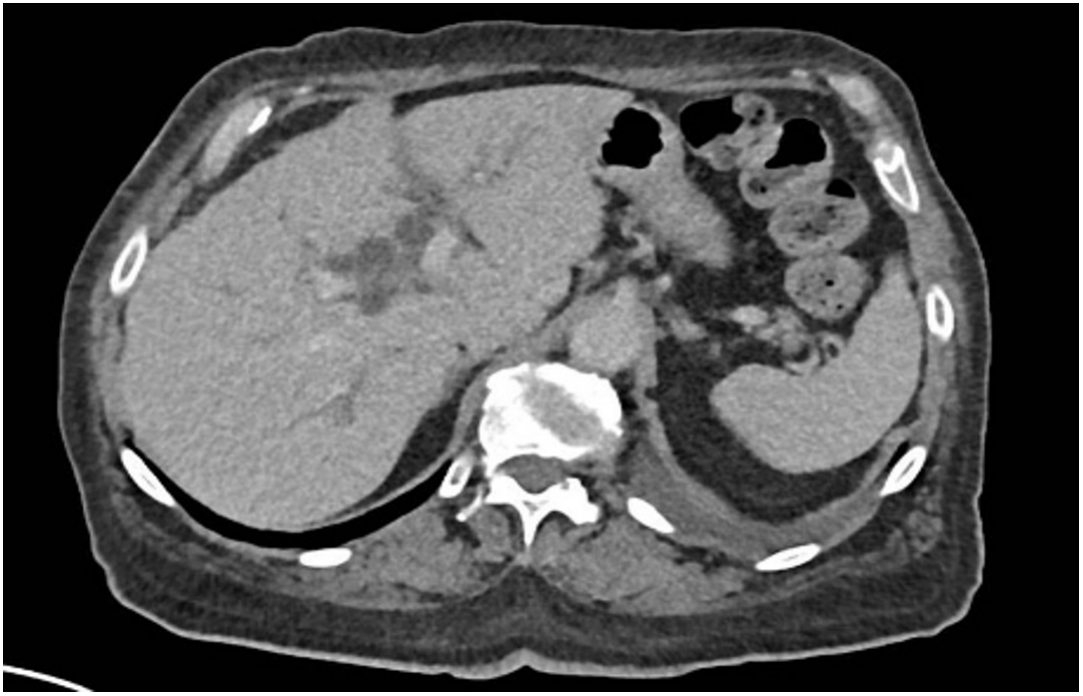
Moderate pleural effusions and mild intrahepatic bile duct dilatation, without clear evidence of progression on Computed Tomography Scan.
